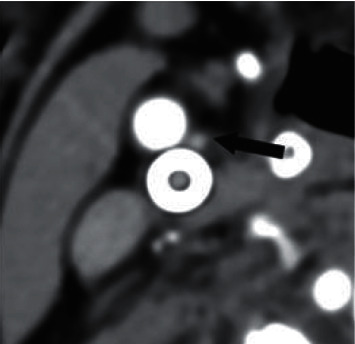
CTA axial slice from an 84-year-old man who had experienced a previous AMI and suffers from HT. The patient also had had a carotid stent implanted, but the enlarged CB (arrow) is still easily identified.
